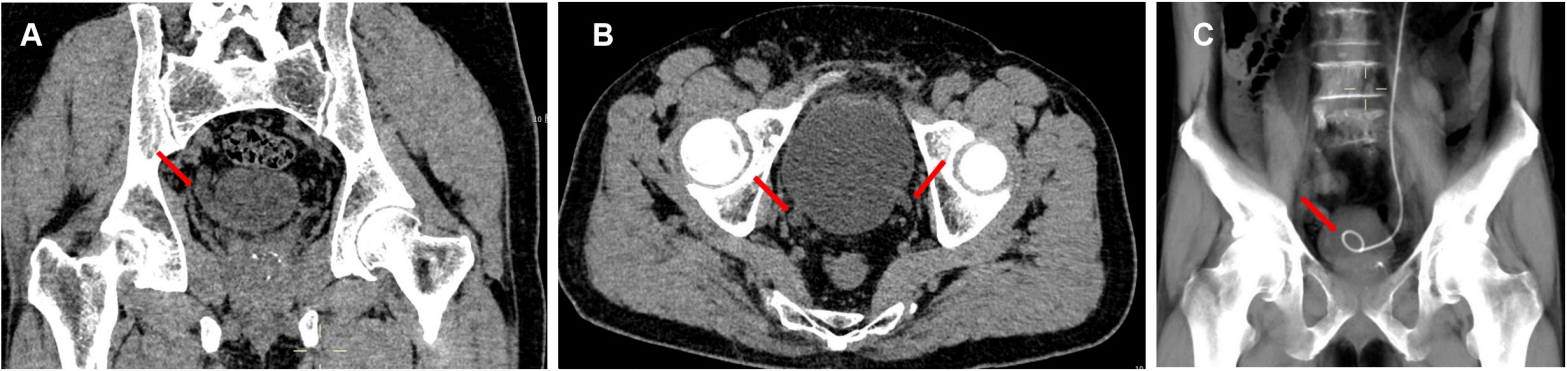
(A) Coronary section of CT examination before PCNL indicated the dilation of right ureter (red arrow). (B) Axial section of CT examination before PCNL showed the dilation of bilateral ureters (red arrows). (C) CT examination showed the location of left ureteral stent after the adjustment guided by cystoscope.
